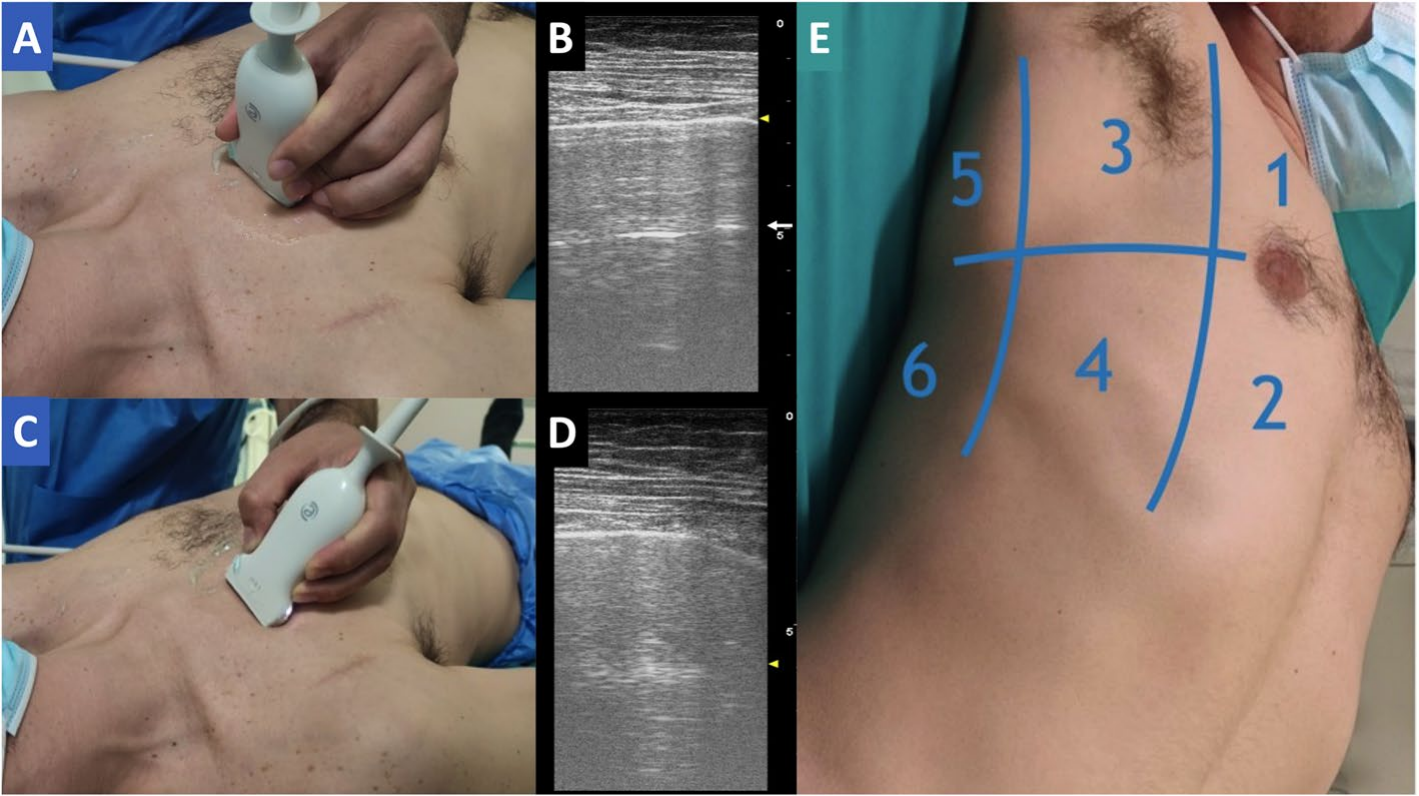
Lung ultrasound. A Transversal scan of an anterior intercostal space: the probe is tilted to be perpendicular to the pleura. In the corresponding ultrasound image (B), the pleural line and one A-line (white arrow) are well visible. One focus is correctly set on the pleura (yellow triangle). C In the same intercostal space, the probe is not correctly tilted and is not perpendicular to the probe. In fact, in the corresponding scan, the A-line is not visible anymore (D); moreover, the focus is set too deep, leading to a less distinct visualization of the pleural line. E The 6 regions per hemithorax of a standard lung ultrasound examination. Anterior fields (1 and 2) are identified by sternum and anterior axillary line, lateral fields (3 and 4) by anterior and posterior axillary lines, posterior fields are accessible below the posterior axillary lines (5 and 6). The patient can lie supine with no need to turn him/her to visualize posterior fields
